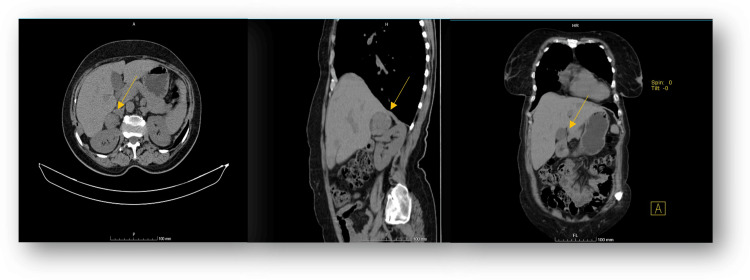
Chest, abdomen and pelvis CT CT scan images (axial, sagittal and coronal view - from left to right) showing a 5x4.4 cm rounded, expansive formation in the right adrenocortical gland (yellow arrows) revealing punctiform parietal calcifications.
